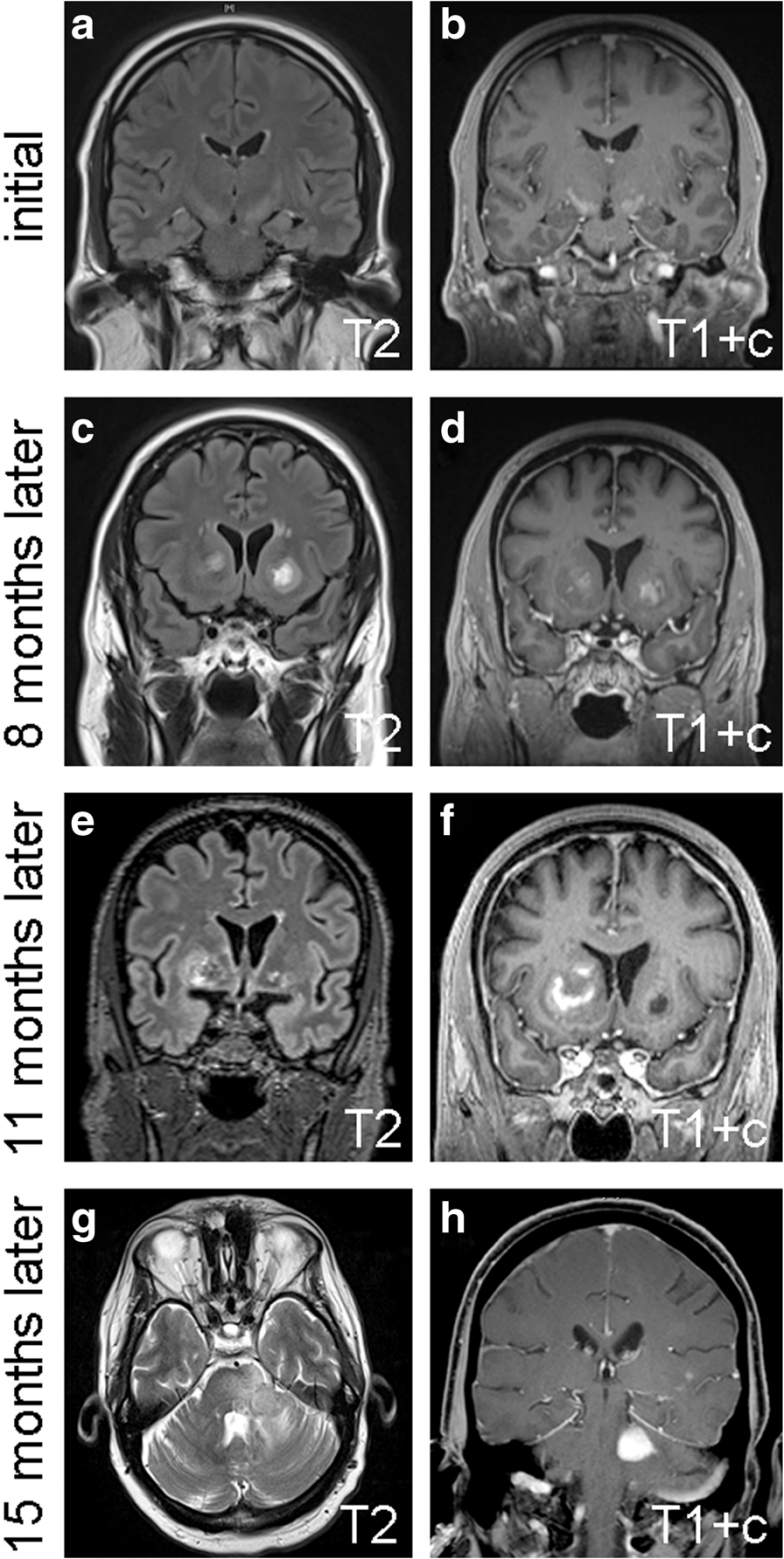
Serial MRIs. The initial set of MRIs showed T2 hyperintensity and some contrast enhancement in the bilateral internal capsules (a, b). Eight months later, a second set of MRIs showed new enhancing lesions in the bilateral basal ganglia (c, d). Eleven months after initial presentation, the right basal ganglia lesion appeared to grow, whereas the lesion in the left basal ganglia diminished (e, f). In the final set of MRI, 15 months after initial presentation, the right basal ganglia lesion was unchanged (not shown), and a new enhancing mass appeared in the left middle cerebellar peduncle (h). c = contrast
